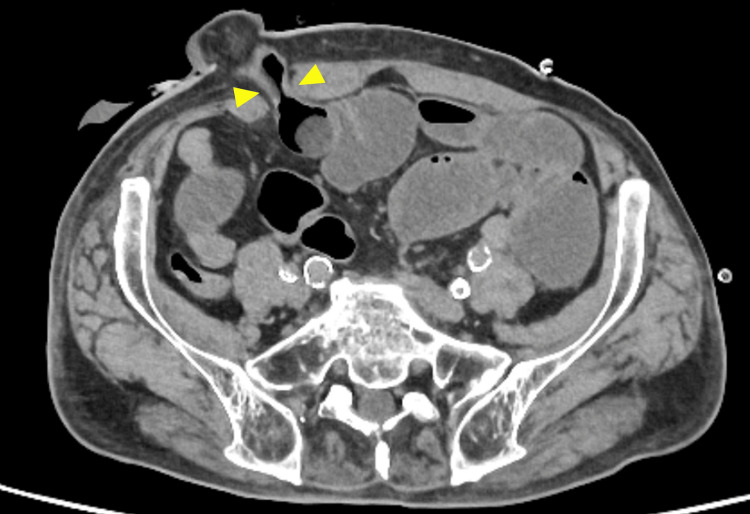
CT image of a small bowel obstruction at the stoma site (axial view) The arrowheads indicate a luminal narrowing of the stoma limb causing small bowel obstruction. Image created by the authors
